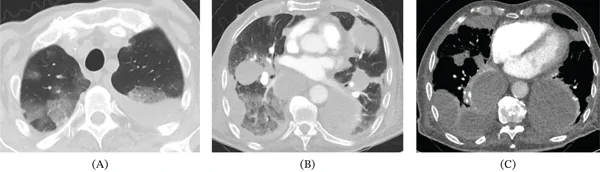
Chest computed tomography (CT) approximately 3 weeks after admission, after repeated bilateral drainage for hemothorax. (A) Worsening ground‐glass opacities in the upper lobes. (B) Marked and rapid progression of the pulmonary nodules, with a loculated pleural effusion on the left side and diffuse interstitial thickening in the right lower lobe. (C) Mediastinal window. Large, multiple, loculated hemorrhagic pleural effusions at both lung bases.
